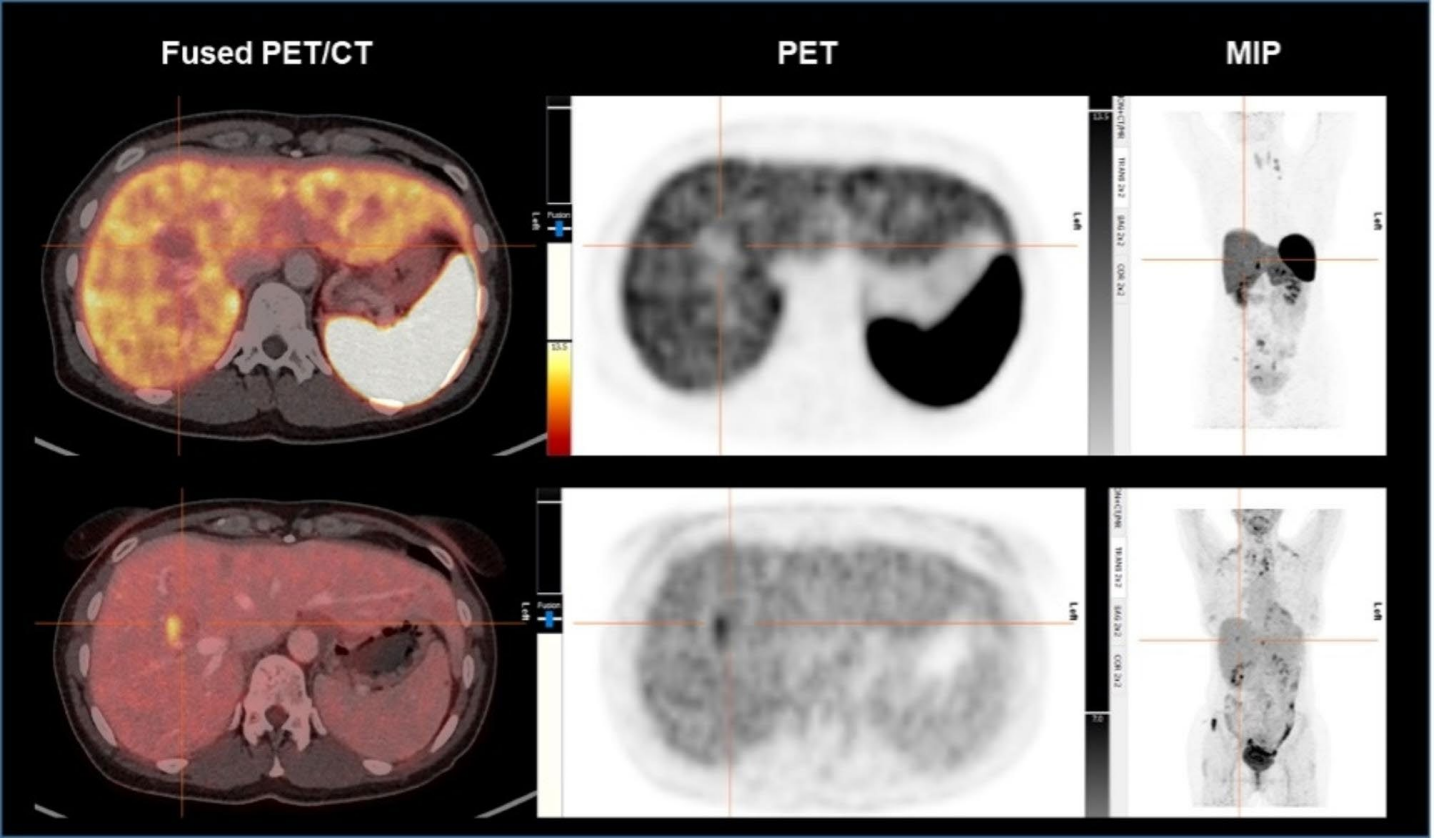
PET/CT of a 47 year old patient with a NET G2 (Ki-67 8%) of the small intestine. Liver lesion in segment 8 with 2.8 × 2.7 cm without [^68^Ga]Ga-DOTATOC-uptake in SSR-PET/CT (first row). [^68^Ga]Ga-DOTATOC-uptake of the small intestine (primary site) led to the suspicion of undifferentiated liver metastases. Additional [^18^F]FDG PET/CT was performed and showed enhanced [^18^F]FDG-uptake (SUVmax 5.8) compared to liver parenchyma. Left images show fused PET/CT (first row: [^68^Ga]Ga-DOTATOC PET/CT, second row: [^18^F]FDG PET/CT, middle images show PET; right images show maximum intensity projections (MIP).
